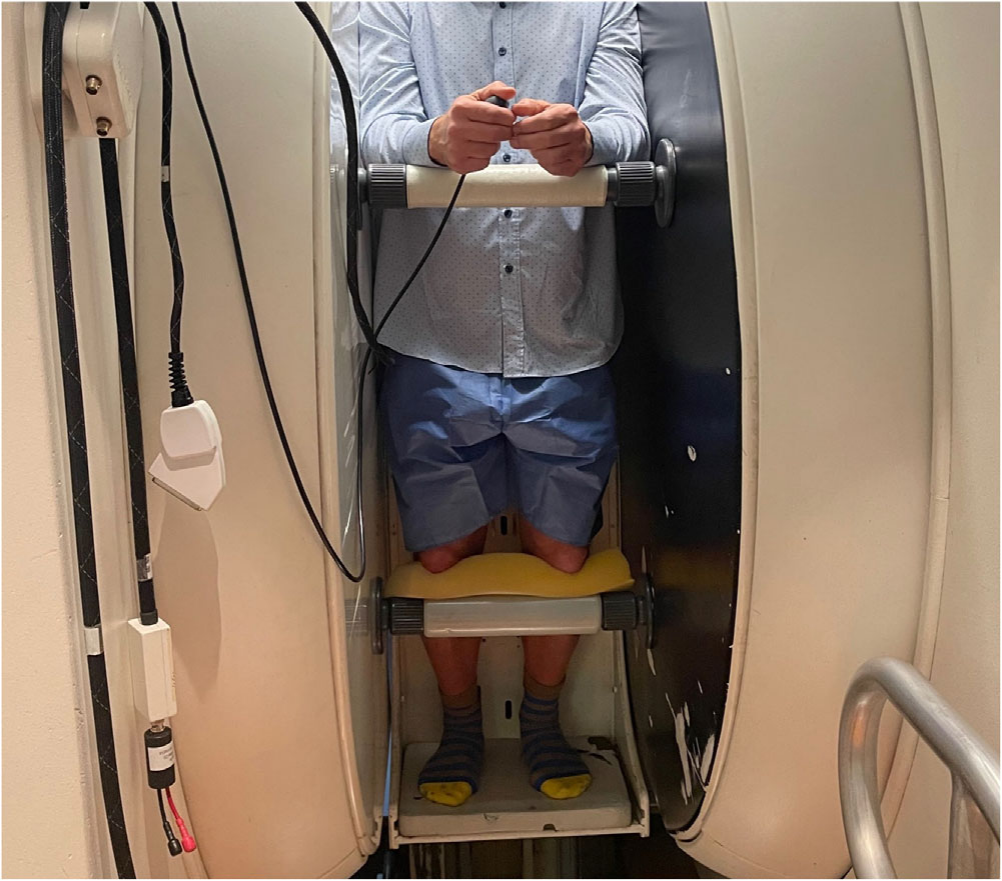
A volunteer in the MRI scanner demonstrating the upright position. Trans‐polar VersaRests™ were positioned to support the knees and arms.
